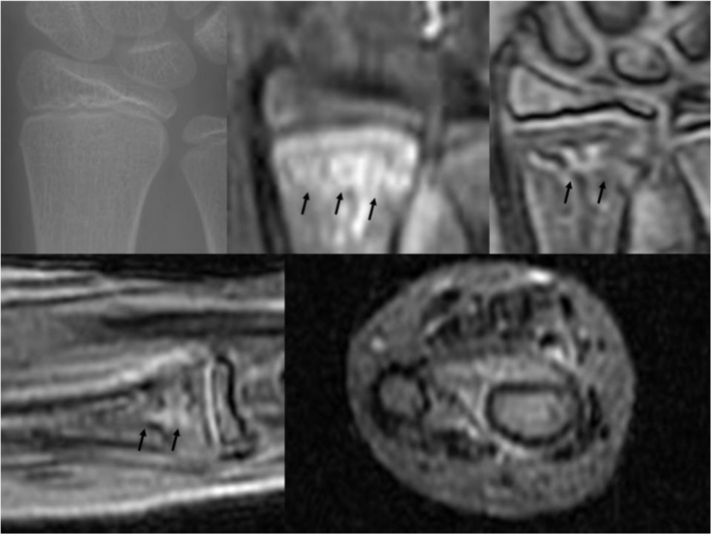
Radiographic, and MRI images of the same patient with Figure
